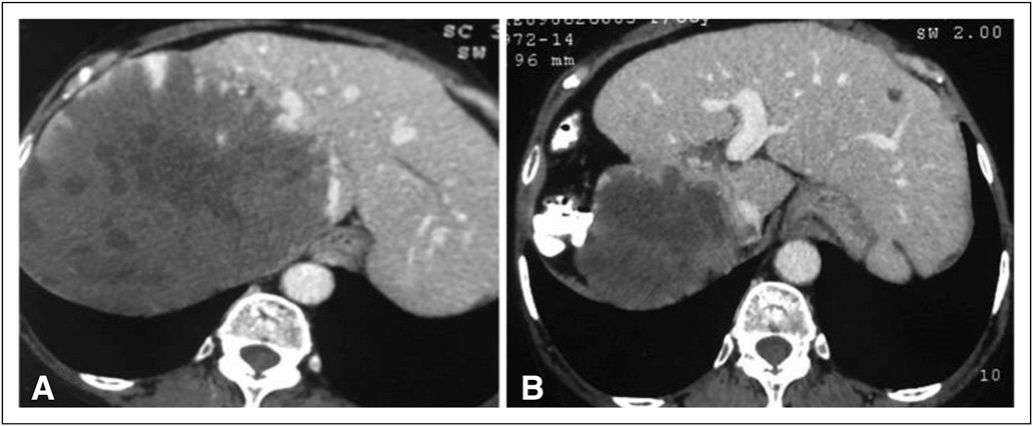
(A) Contrast computed tomography scan of the abdomen of a 65-year-old woman
